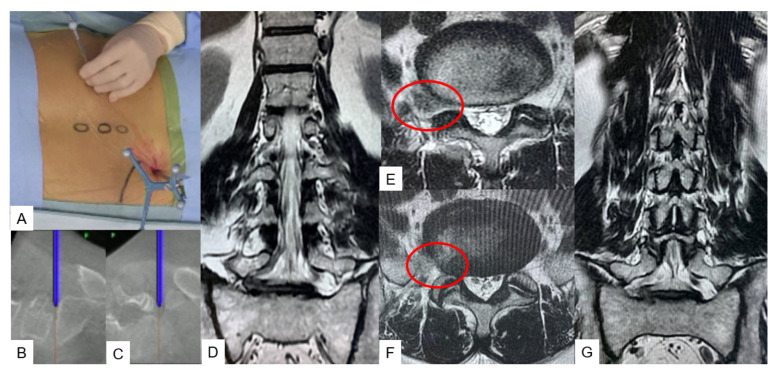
Case 7, 55 M, right L5/S foraminal disc herniation, transforaminal discectomy with navigation, (A) Intraoperative image, (B) Sagittal navigation image, (C) Coronal navigation image, (D) Preoperative coronal T2 weighted MR imaging, (E) Preoperative axial T2 weighted MR imaging, (F) Postoperative axial T2 weighted MR imaging, (G) Postoperative coronal T2 weighted MR imaging.
